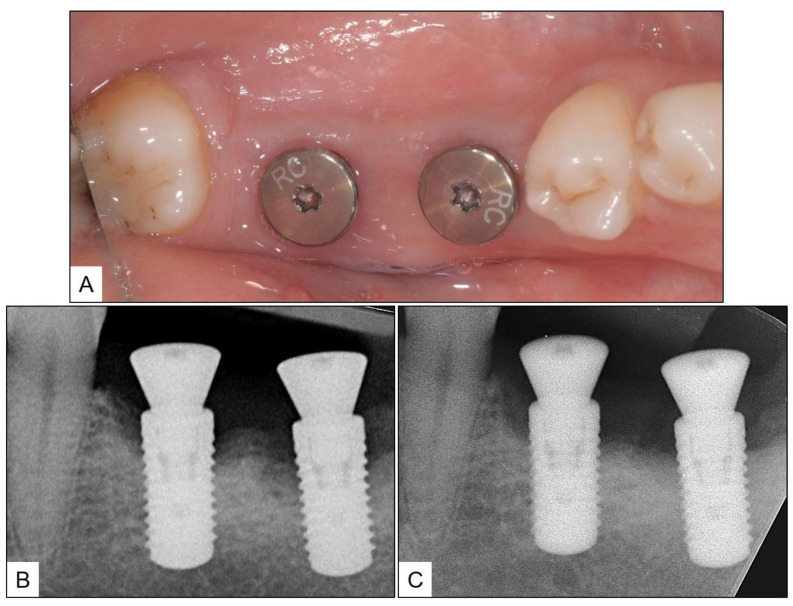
Follow-up clinical photograph after 3 months and comparison of radiographic bone height showing (A) Complete clinical resolution of peri-implantitis; 3 months after the last session of non-surgical therapy and local oxygen-based therapy with blue^®^m products; (B) Pre-treatment periapical radiograph showing peri-implant bone loss; and (C) Post-treatment periapical radiograph showing significant improvement in peri-implant bone height.
